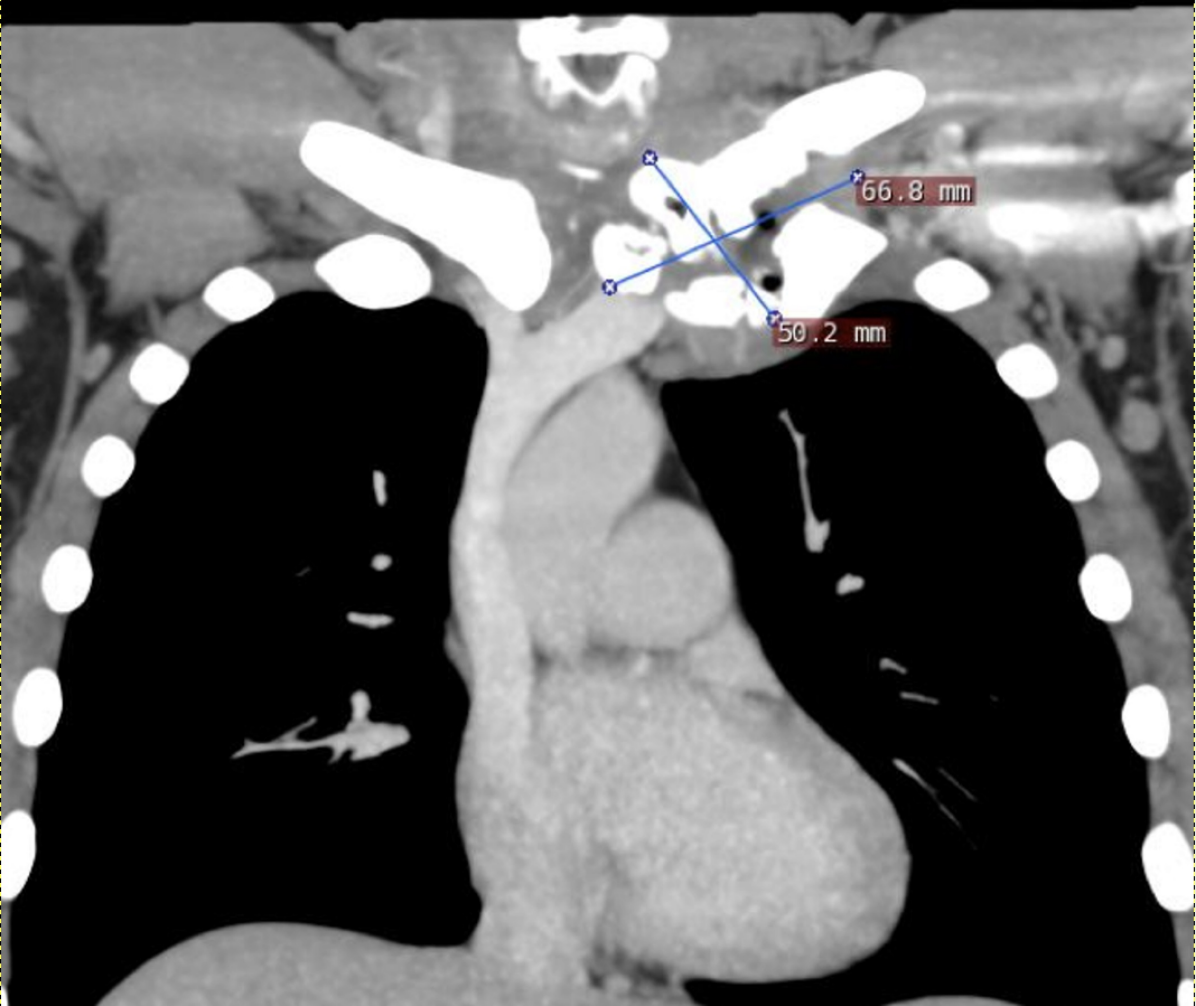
Coronal CT Chest A coronal CT of the chest with IV contrast demonstrating destructive changes at the left SCJ and first costochondral junction with fluid collection and air measuring up to 6.5 cm consistent with septic joint and osteomyelitis.
